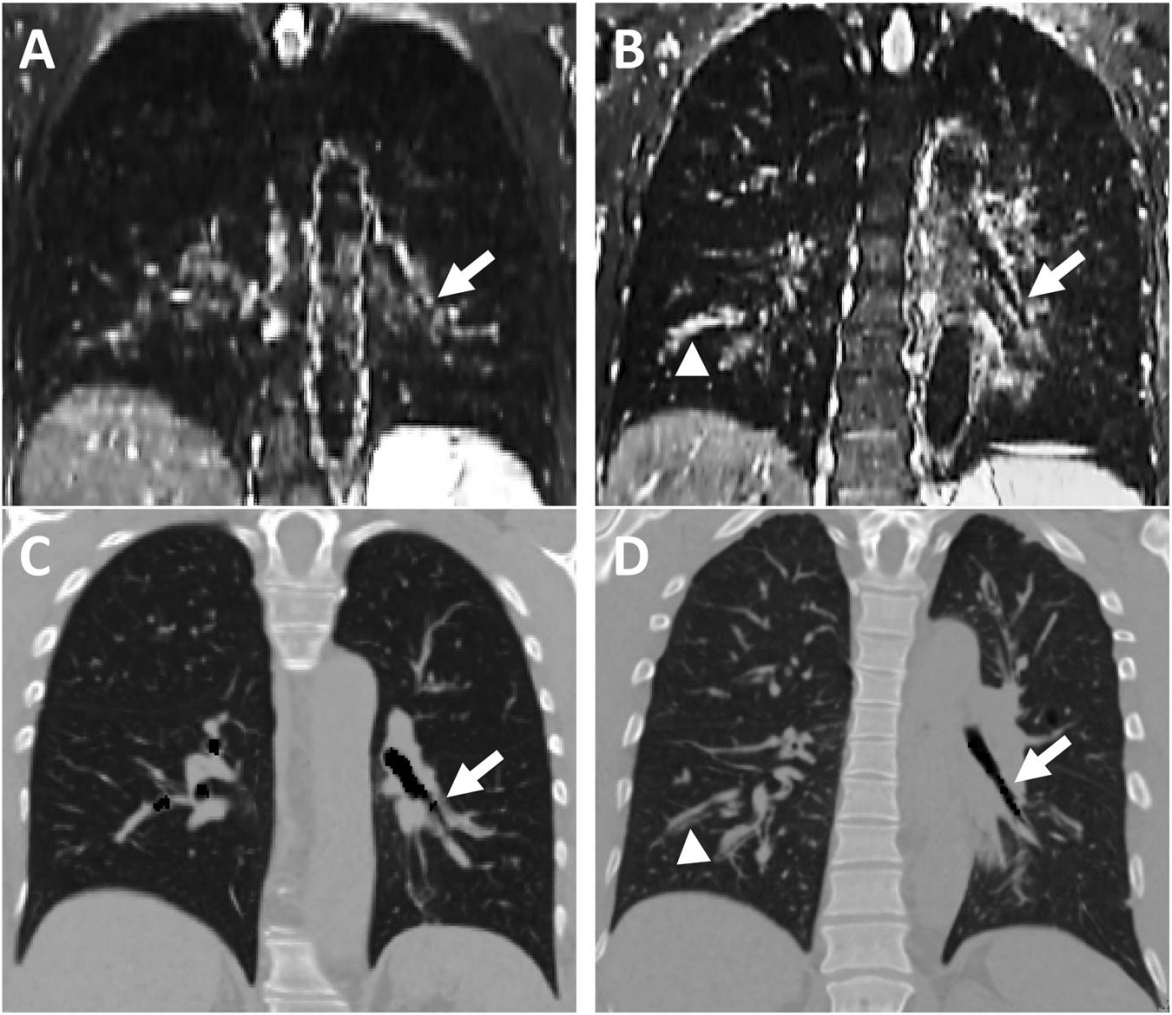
Coronal plane of registered T2w MRI (A, B) and registered CT (C, D) of a 48-year-old non-severe asthmatic female patient (left panels) and a 47-year-old severe asthmatic male patient (right panels). White arrows show the left lower lobe bronchus. Note a higher bronchial wall intensity signal on T2w images in severe asthmatic patient (B) in comparison to non-severe asthmatic patient (A) also indicated by the white arrowhead in sub segmental bronchus of the right lower lobe (B). BrWall_T2-MIS = 63 and 93 in non-severe and severe asthmatic patients, respectively
